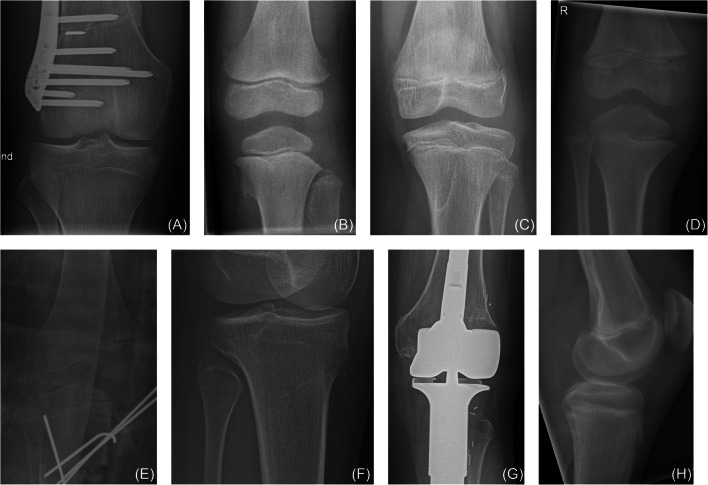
Cropped knee radiographs for three patients. The upper row depicts radiographs that were included into the study while the lower row shows examples of radiographs that were excluded. A Male patient (18.5 years). B Female patient (3.3 years). C Female patient (13.0 years). D Male patient (6.8 years). E Female patient (15.7 years), excluded because of low image quality. F Female patient (5.3 years) excluded because the knee is not fully visible. G Female patient (19.8 years) excluded because of knee arthroplasty. H Male patient (16.5 years) excluded because of lateral view
